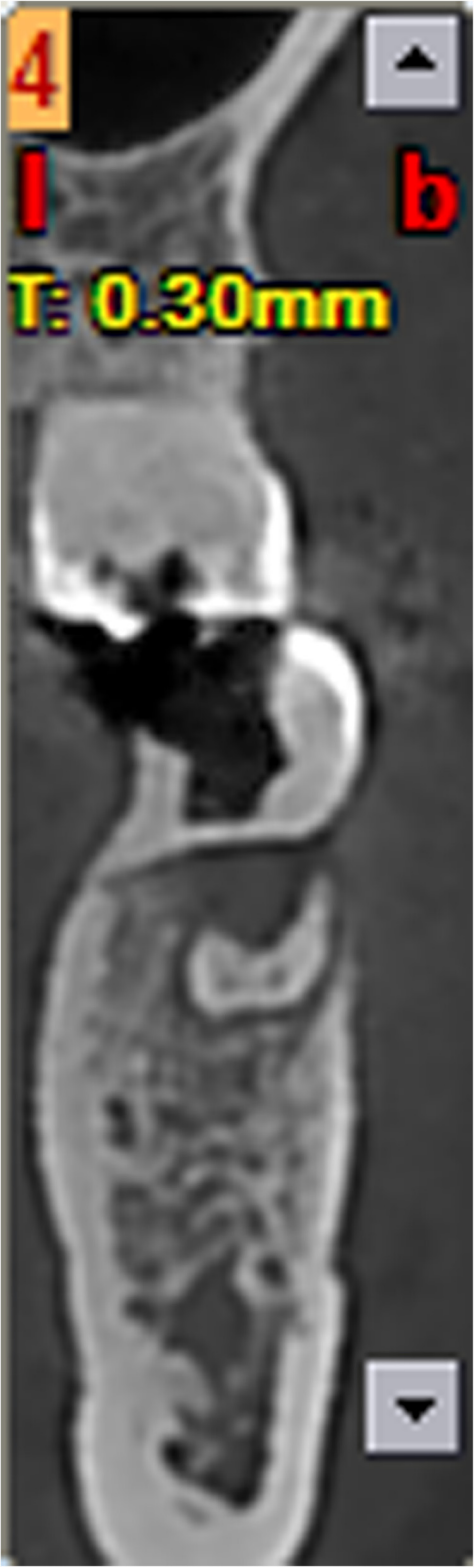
Computed tomography buccolingual section view.
